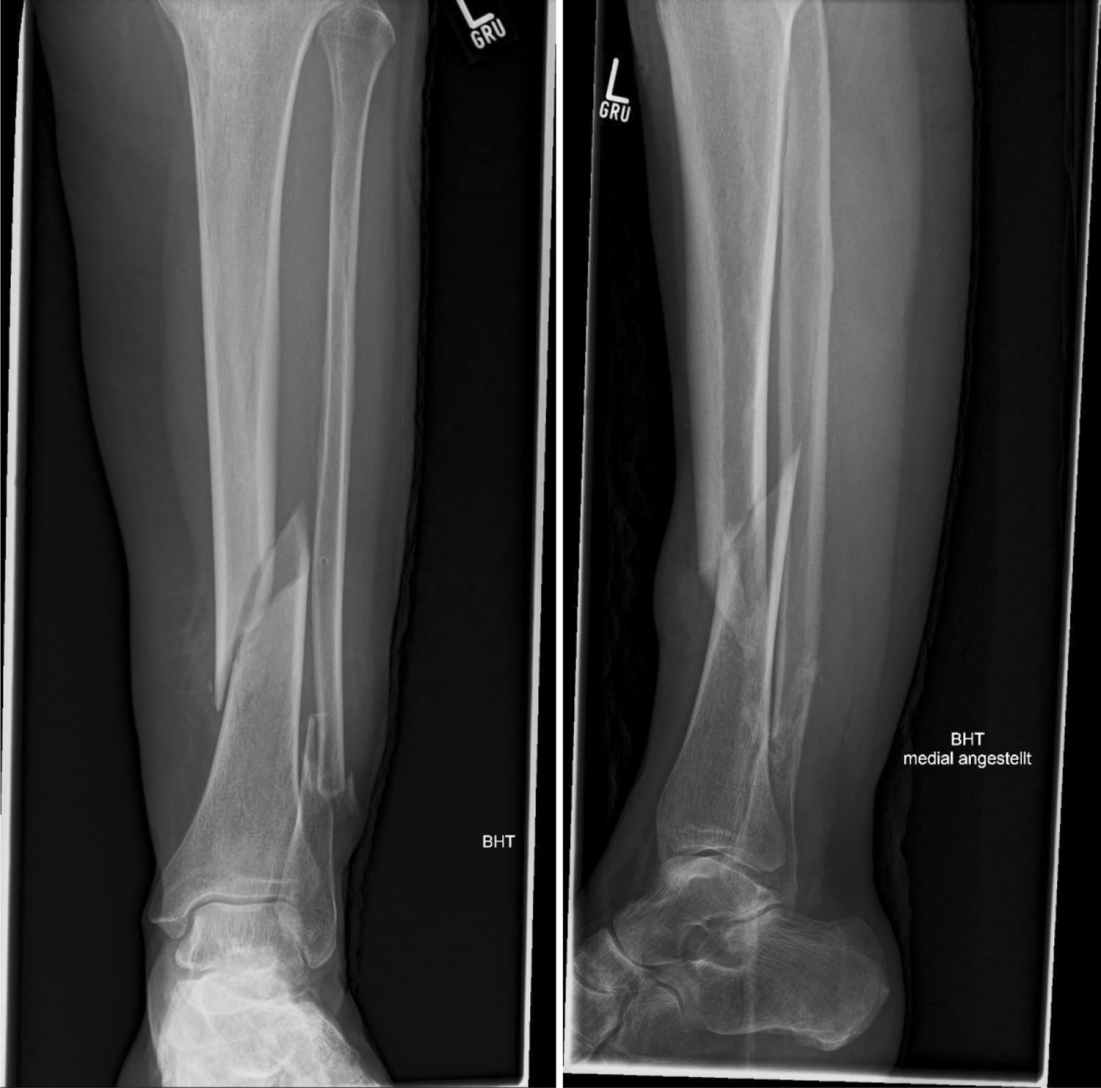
X-ray of a spiral fractue of the lower leg (AO 42A1c/4F2B) in ap and lateral view
